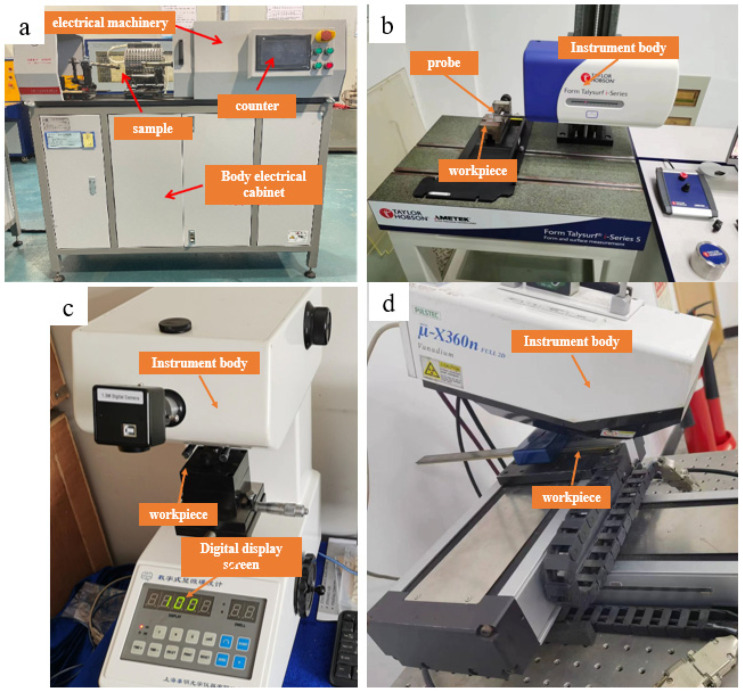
(a) QBWP-1000 cantilever bending fatigue testing machine; (b) New View 5022 surface profiler; (c) automatic microhardness tester; (d) μ-X360n X-ray residual stress analyzer.
